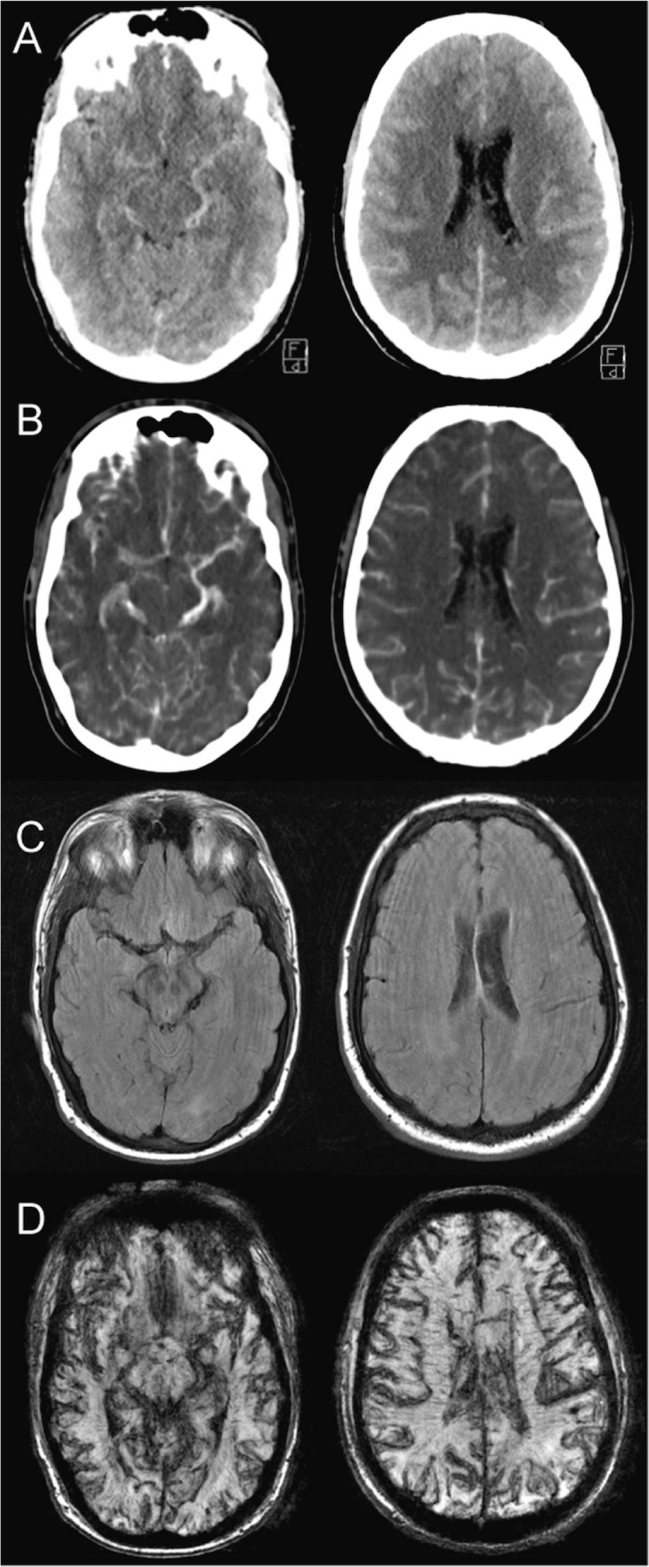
Imaging. Exemplary images of matching slice plains. All images represent 5 mm slices, with images in A, B and D being reconstructions of thinner source images. MR images are of reduced quality due to motion artefacts. A: Non-enhanced CT: Diffuse subarachnoid hyperattenuations mimicking SAH. B: CT-angiography: Sulcal and basal veins dilated and increased in number. C: MRI, FLAIR sequence: No findings of SAH. D: MRI, SWAN sequence: Markedly hypointense depiction of cerebral and subarachnoidal veins due to hypoxaemia.
